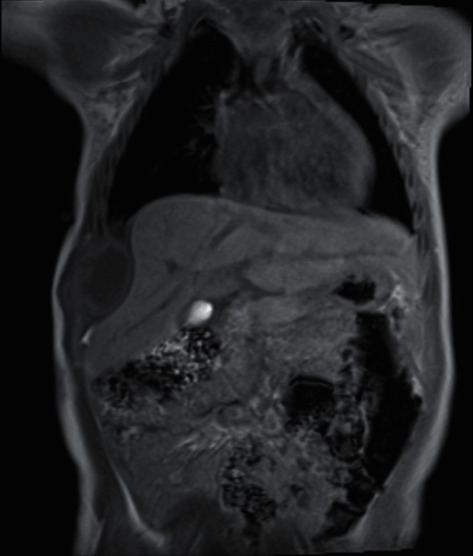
Chest MRI shows lobulated irregular rim-enhancing lesion epicenter with destruction of the right lateral 8^th^ rib causing pressure effect to right hepatic lobe. Penumbra sign is positive.
